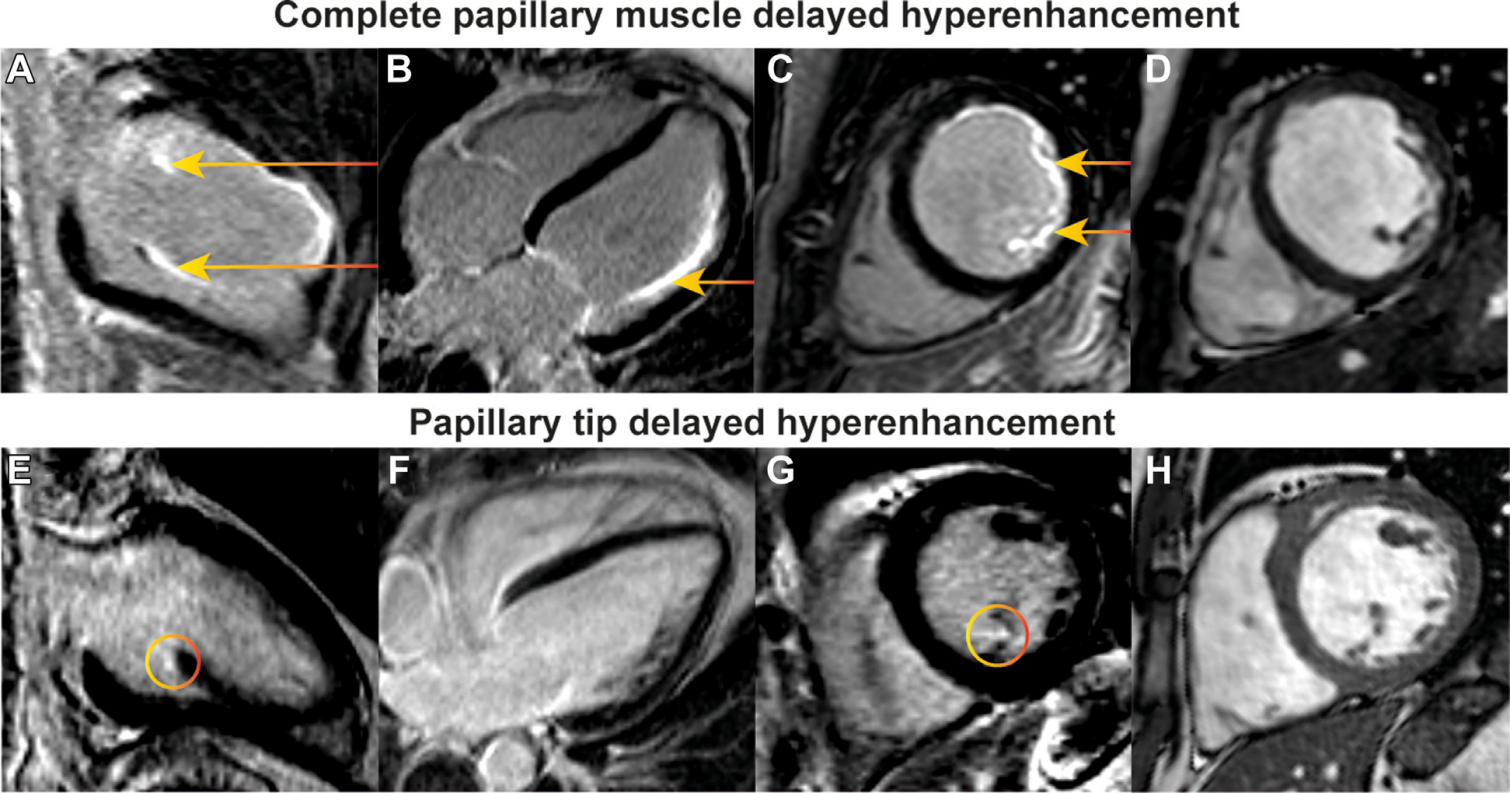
Delayed Hyperenhancement of the Papillary Muscle and Papillary Muscle Tip Delayed hyperenhancement of the papillary muscle vs the papillary tip (A to D) are respectively 2-chamber LGE CMR images, 4-chamber LGE CMR images, short-axis LGE CMR images, and short-axis end-diastolic cine frames of a patient with marked papHE. (E to H) The same frames of a patient with HE of the papillary tip only. The arrows mark papHE and the circles papillary tipHE. CMR = contrast-enhanced cardiac magnetic resonance imaging; HE = delayed hyperenhancement; LGE papHE = papillary muscle delayed hyperenhancement.
